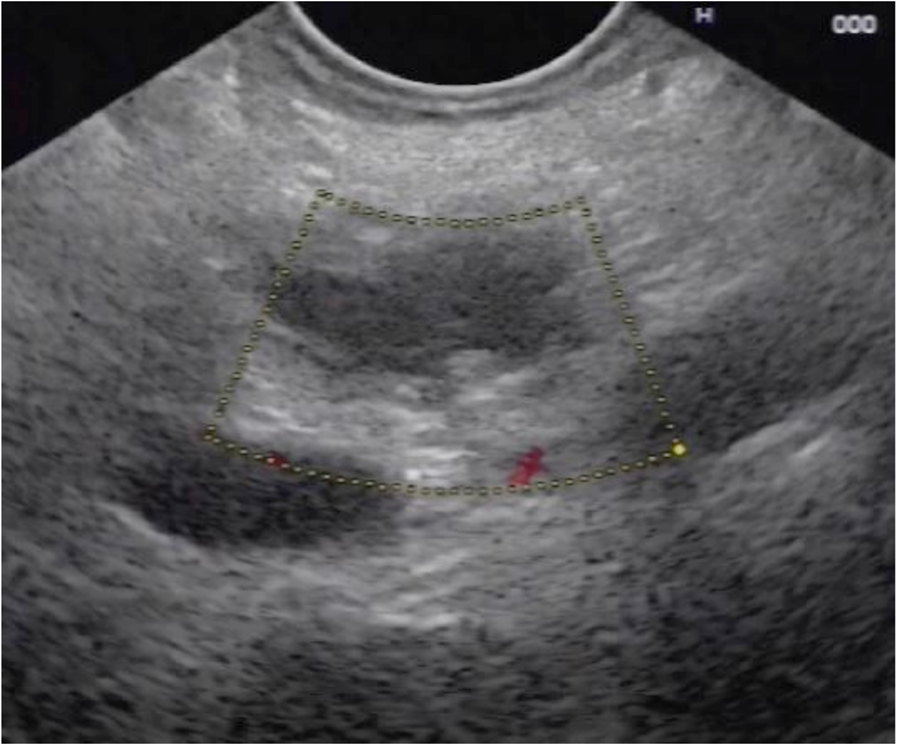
Transgastric endosonography reveals a dilation of the pancreatic duct to a diameter of approximately 7 mm
